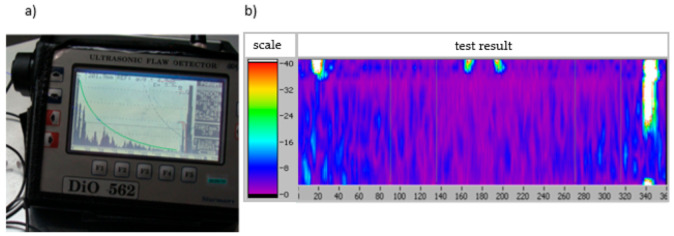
Example of the results of: (a) ultrasonic inspection and (b) eddy current inspection, indicating the presence of cracks in an aluminium casting.
